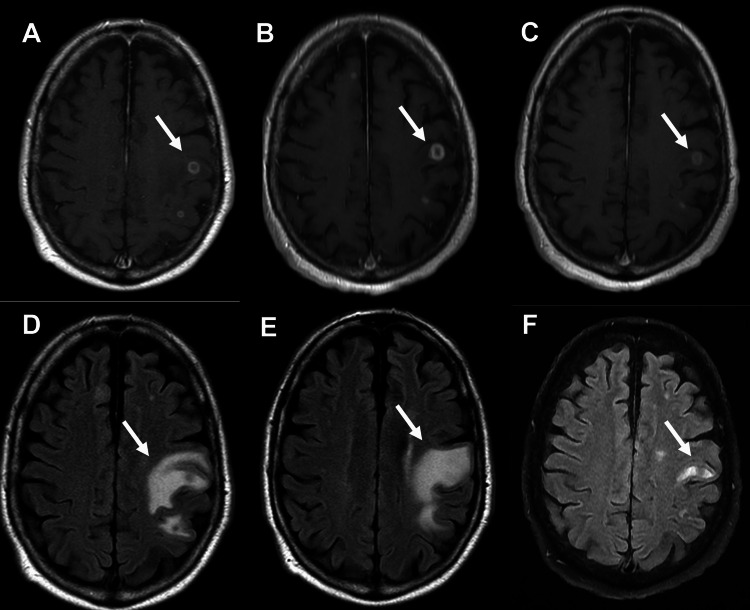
Imaging showing lesions and edema in the left precentral gyrus before and after treatment initiation. (A) Initial axial T1 with contrast MRI showing multifocal ring enhancing lesions with associated vasogenic edema with the largest lesion shown by the white arrow. (B) Axial T1 with contrast MRI showing multifocal ring enhancing lesions with associated vasogenic edema with progression of size/edema on imaging. This image is four weeks after presentation without any treatment. Arrow indicates the largest lesion. (C) Axial T1 with contrast MRI showing multifocal ring enhancing lesions with associated vasogenic edema, with reduction in size and edema, four months after treatment initiation. Arrow indicates the largest lesion. (D) Axial T1 flair on initial presentation, showing vasogenic edema as indicated by the arrow. (E) Axial T1 flair showing progression of vasogenic edema, four weeks after presentation without any treatment. Arrow indicates edema from largest lesion. (F) Axial T1 flair four months after starting treatment, showing reduction in vasogenic edema. Arrow indicates reduced edema from the largest lesion.
